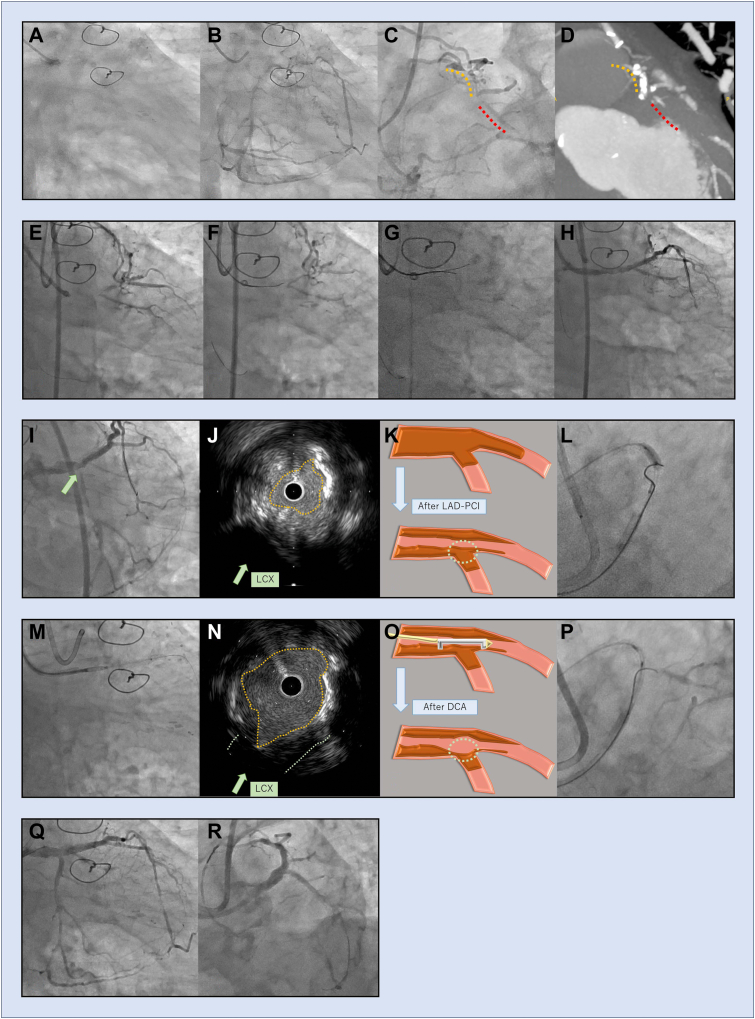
(A-D) Pre-coronary angiography and cardiac computed tomography showed left main artery chronic total occlusion involving “disconnected bifurcation” (Videos 1-3, view videos online). (E-H) Left anterior descending artery percutaneous coronary intervention (LAD PCI). Initial antegrade wiring with Gaia Next 3 (Asahi Intecc, Nagoya, Japan) failed to penetrate the distal cap. A parallel wire technique using Confianza Pro 12 (Asahi Intecc) successfully crossed the lesion. (I-L) After LAD PCI, intravascular ultrasound revealed that the entry was covered by thick plaque, and even Confianza 8-20 (Asahi Intecc) could not penetrate the cap (Video 4, view video online). (M-P) After directional coronary atherectomy (DCA; Video 5, view video online), intravascular ultrasound confirmed a reduction of the plaque covering the cap, and the Confianza 8-20 successfully penetrated the cap (Videos 6 and 7, view videos online). (Q, R) Final coronary angiography (Videos 8 and 9, view videos online). LCX, left circumflex artery.
